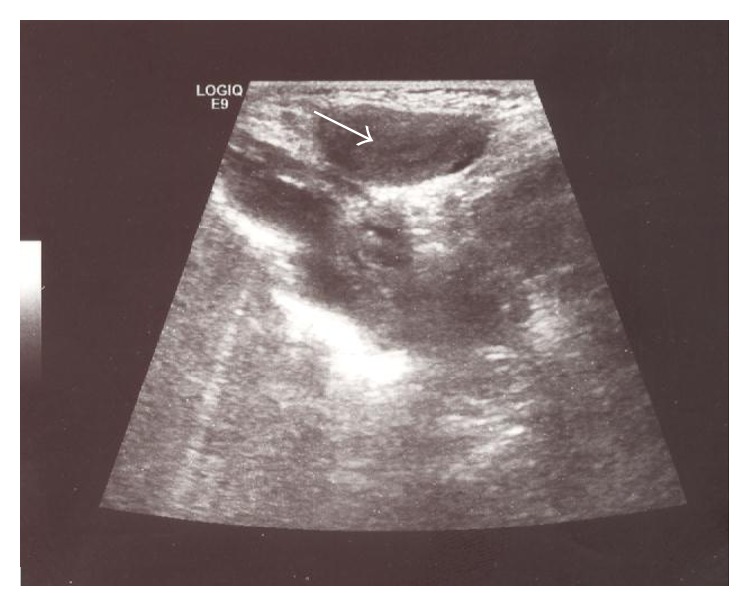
Ultrasonography image: a solid mass in right inguinal channel with a clearly visible endometrial lining.
